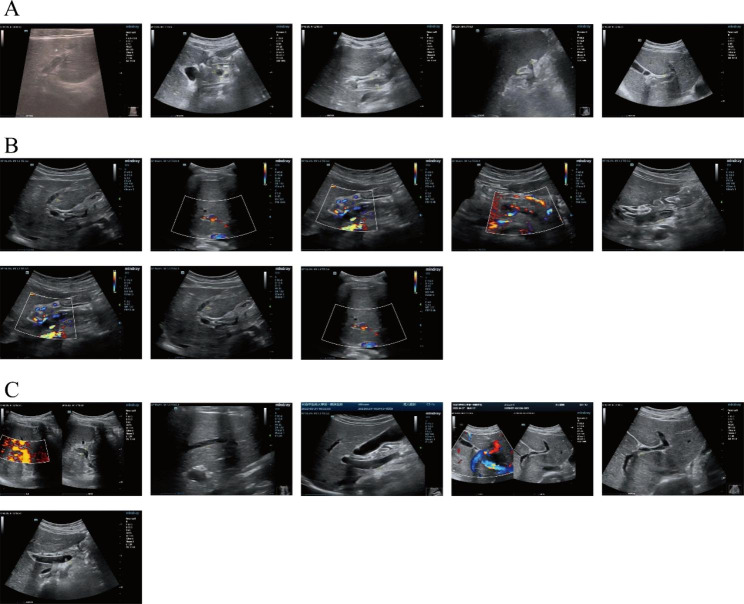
Thromboses of portal, splenic and superior mesenteric veins. A represents the Doppler ultrasound test on admission. B represents the Doppler ultrasound after 10 days. C represents the Doppler ultrasound test after 25 days
